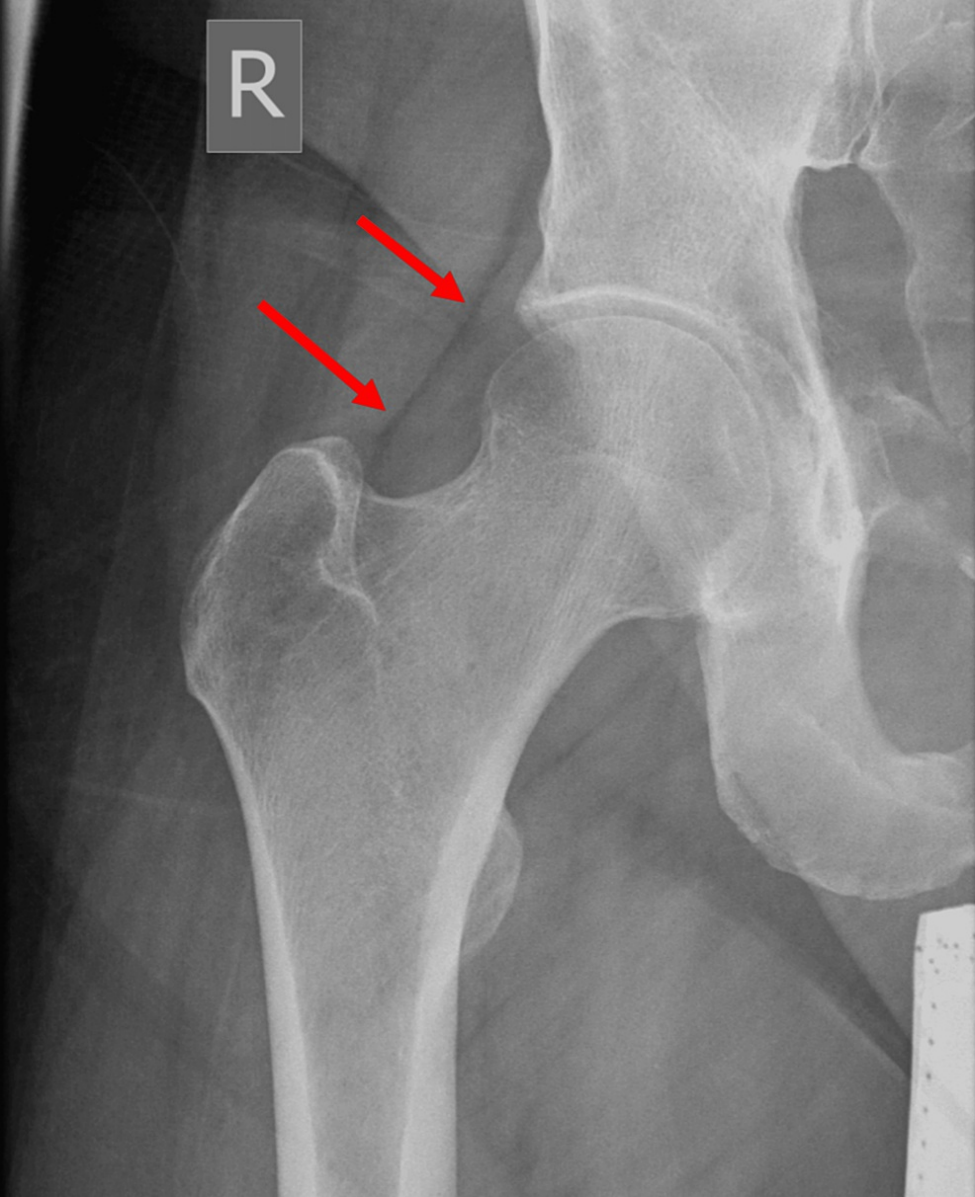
Radiograph of the right hip. Right-sided hip effusion (arrows).
